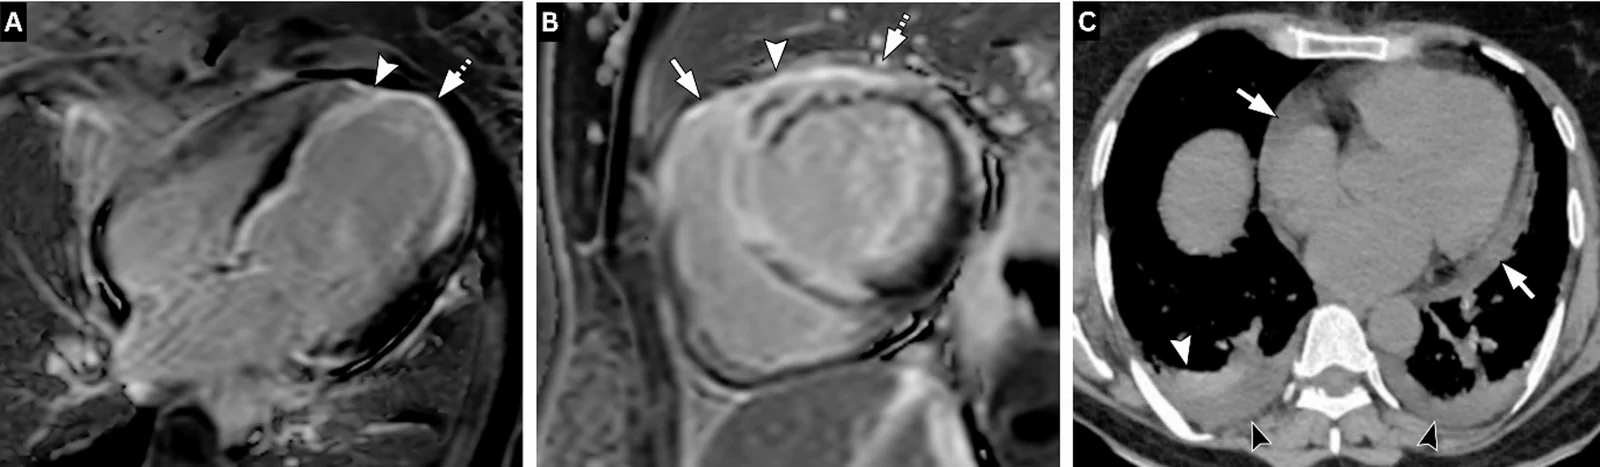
A, B Myocarditis in a 35-year-old female with scrub typhus presenting with fever, bradycardia, and lower-limb edema. Cardiac MRI four-chamber SSFP (A) and short-axis SSFP (B) demonstrate epicardial-to-mid myocardial late gadolinium enhancement of the left ventricle (white dotted arrows), with additional involvement at the right ventricle (RV) insertion points (white arrowheads) and along the RV free wall (solid white arrow). C Pericardial effusion in a 62-year-old female with scrub typhus and pneumonia presenting with fever and cough for 5 days and breathlessness for 1 day. Axial CT chest (C) shows a small volume pericardial effusion (solid white arrows). Also seen are bilateral small-volume pleural effusions (black arrowheads) and passive atelectasis of the adjacent right lower lobe (white arrowhead)
